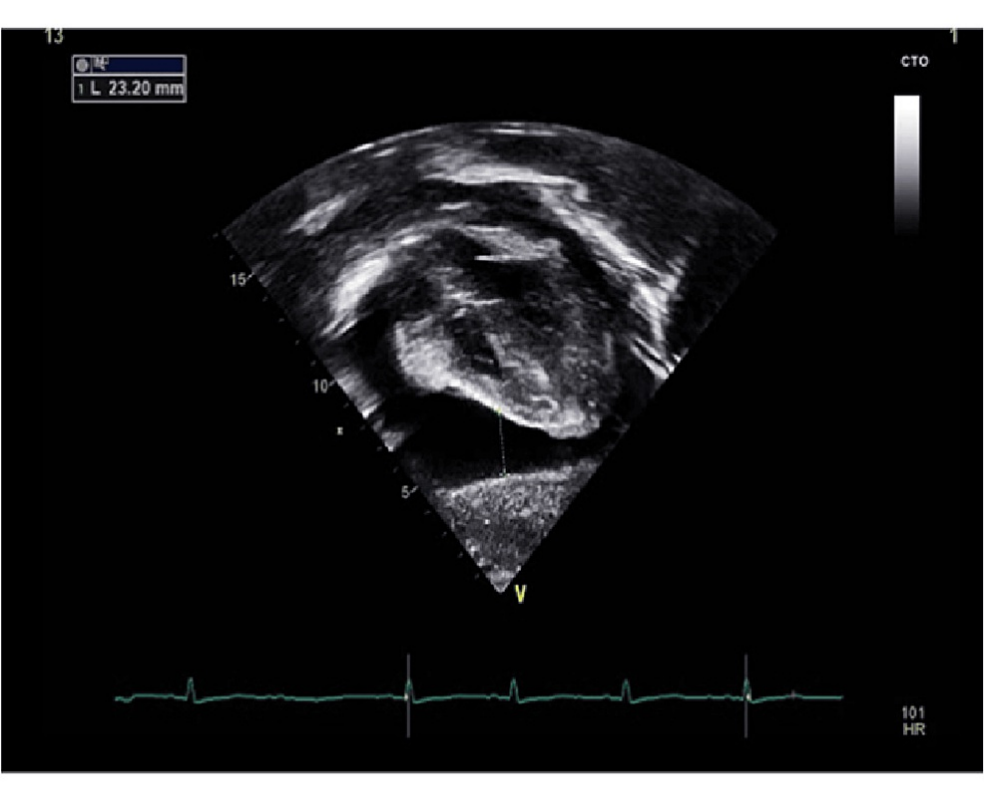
Echocardiogram demonstrating a large amount of pericardial effusion measures more than two centimeters
